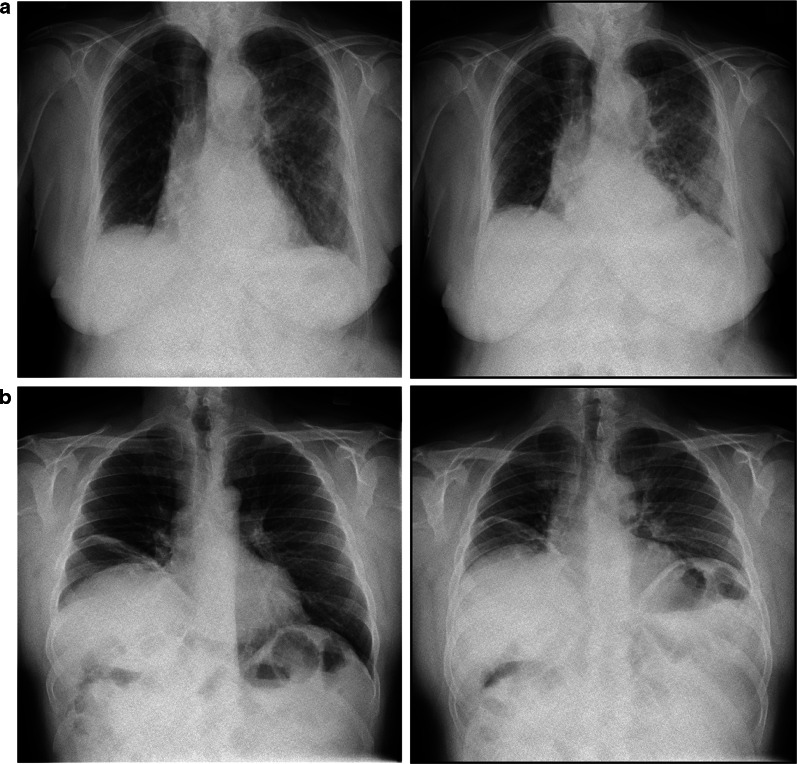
Demonstration of use of dynamic chest radiography in lung parenchymal and diaphragmatic disorders. Still frame at full inspiration shown on left, and still frame at expiration on the right. a DCR post-left lower lobectomy complicated by pneumonia, in a female in her 70 s, demonstrating left mid- and lower-zone consolidation, with normal diaphragmatic movement. b DCR in a male in his 50 s, demonstrating an elevated right hemidiaphragm; paradoxical right diaphragm movement on sniffing consistent with phrenic nerve palsy
